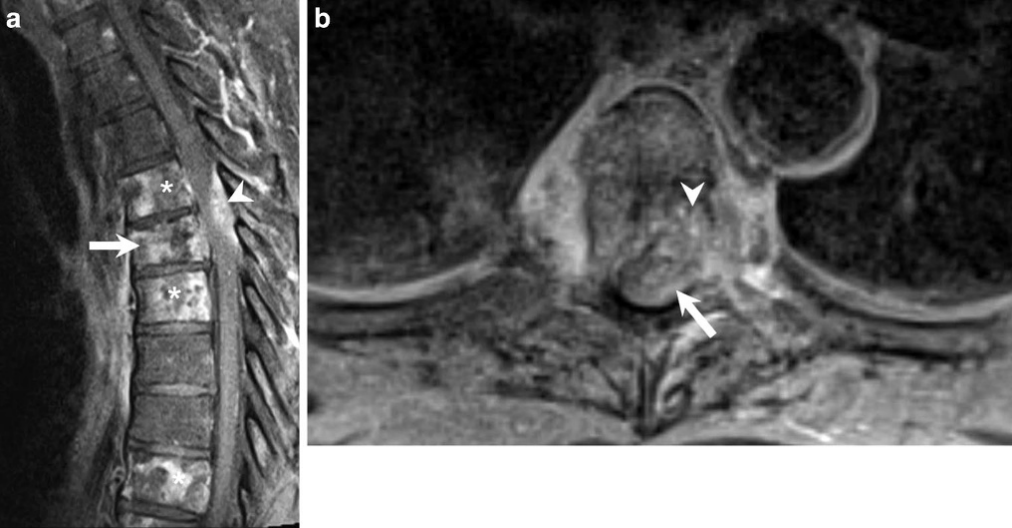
Sagittal T1-weighted images after administration of gadolinium (a) showed a marked yet patchy pattern of enhancement of the affected vertebral bodies (*arrow* and *asterisks*). The epidural lesion exhibited homogeneous contrast enhancement and displaced the myelon ventrally (*arrowhead*). On axial T1-weighted postcontrast images (b) the epidural portion of the lesion compressed the myelon (*arrow*), and the infiltration of the vertebral body (*arrowhead*) could be appreciated
